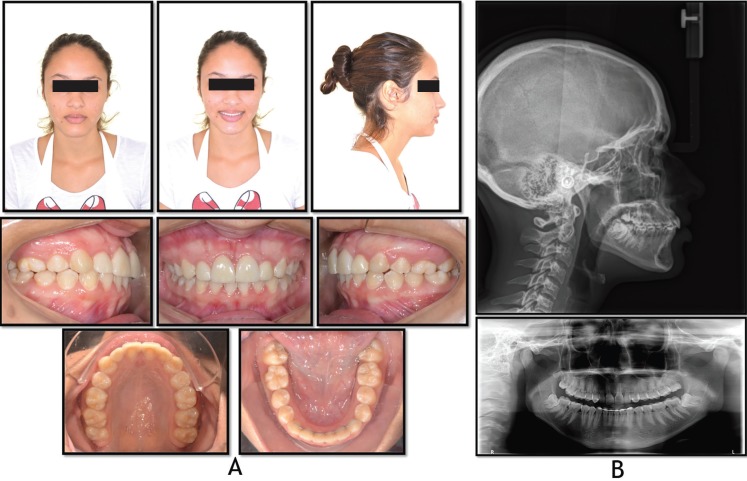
Posttreatment: A) facial and intraoral photographs; B) cephalometric and panoramic radiographs.
